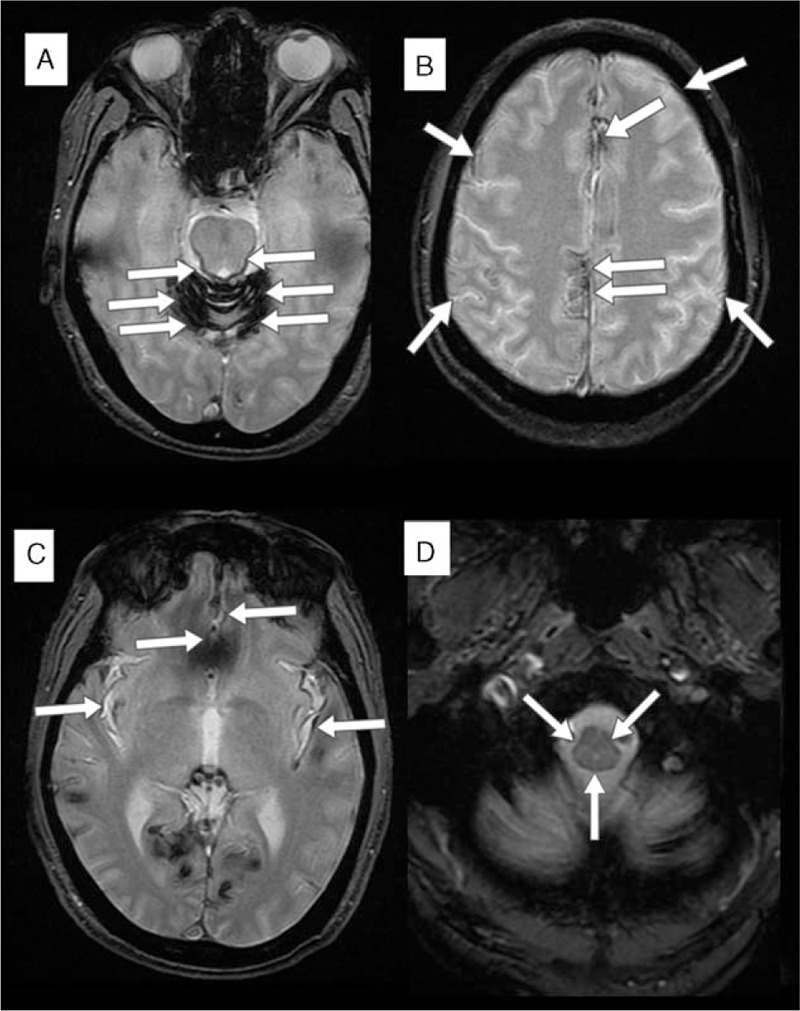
Axial T2-weighted brain magnetic resonance imaging showed diffuse hypointensity signals (white arrows) along with the cerebrospinal fluid spaces, most severe in the cerebellar folia (A), and also around pons (A), higher interhemispheric fissure (B), lower interhemispheric fissure and Sylvian fissure (C), and spinal cord (D).
